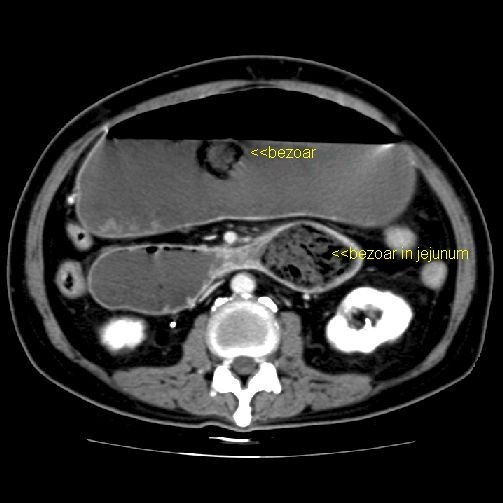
CT image of trichobezoar. [K M. Vitellas, K Vaswani and W F. Bennett: Small-Bowel Bezoar *AJR *2000; 175:876–878].
